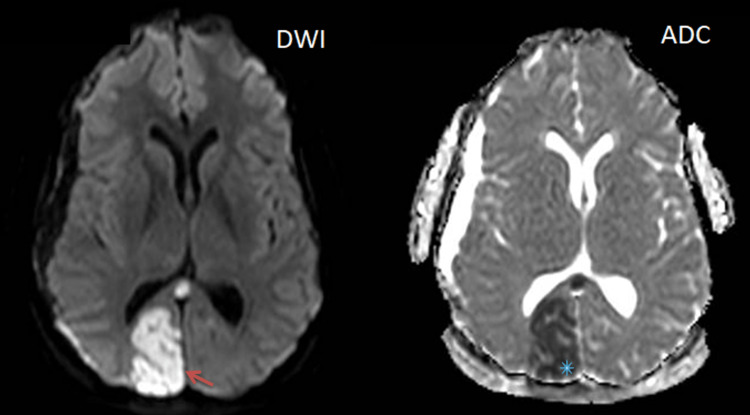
Acute ischemic stroke of right PCA territory, in addition to chronic right subdural hematoma. MRI (transverse view) showing the area of restricted diffusion in the medial aspect of the right occipital lobe that appears hyperintense on DWI (red arrow) and hypointense on ADC mapping (asterisk), with a right-sided subdural collection that follows CSF. PCA: posterior cerebral artery, MRI: magnetic resonance imaging, DWI: diffusion-weighted imaging, ADC: apparent diffusion coefficient, CSF: cerebrospinal fluid.
